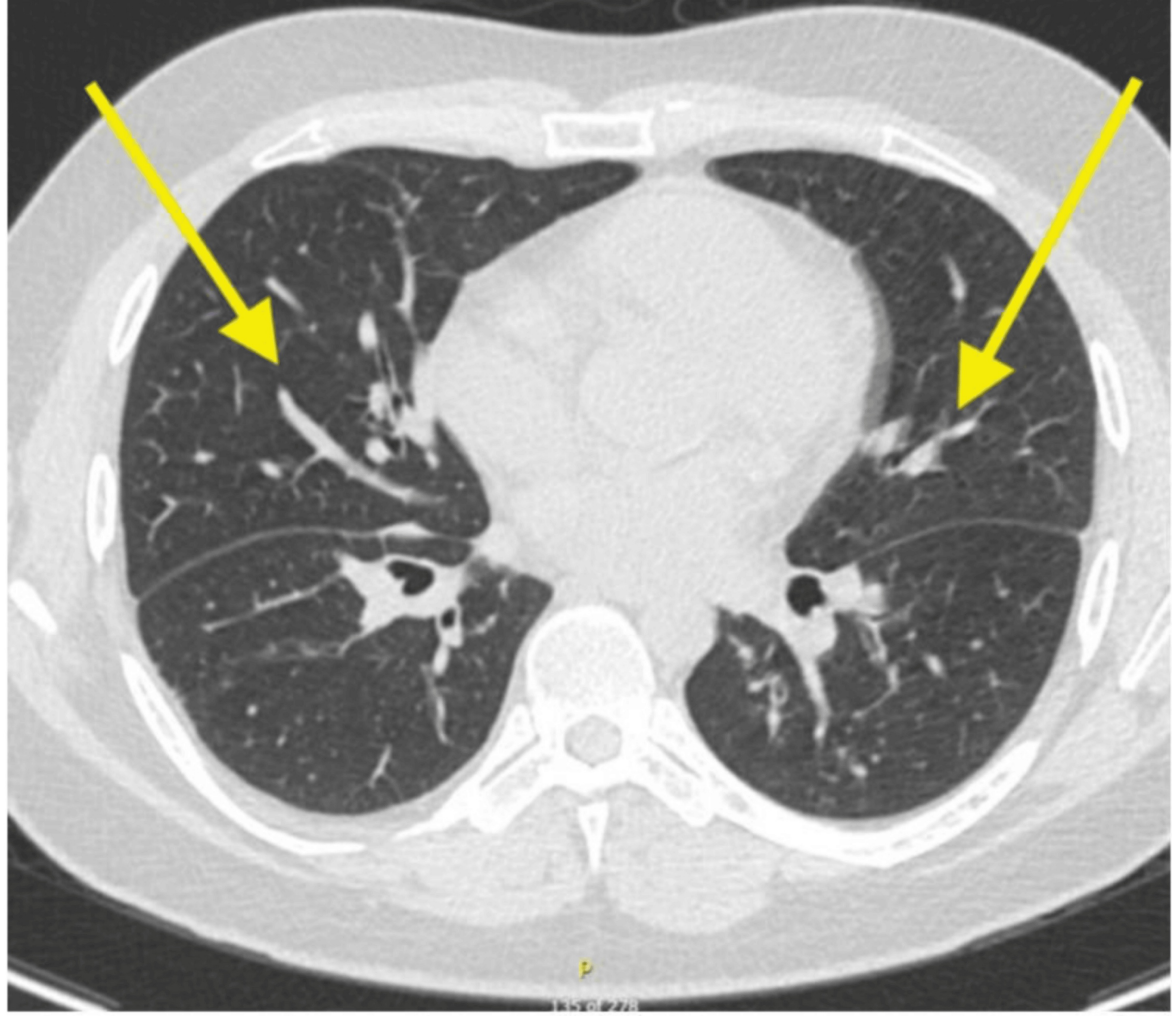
Chest high-resolution CT at the 6-month follow-up showing diffuse bronchial wall thickening (yellow arrows) and nearly resolved faint centrilobular ground-glass attenuation
